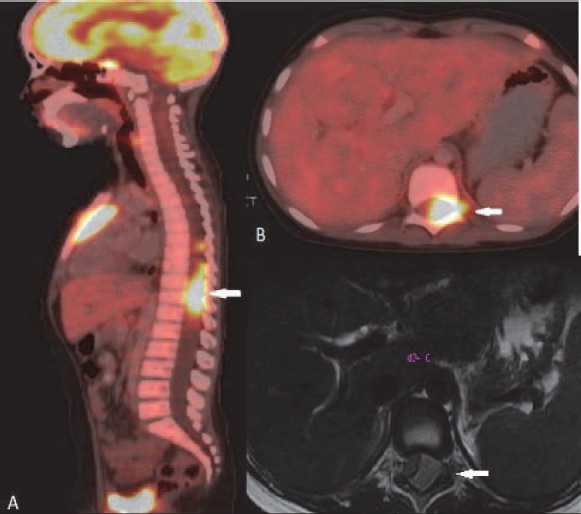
Same patient as in Figure 19. (A) Sagittal fused PET/CT image shows FDG avid intraspinal deposit. Axial fused PET/CT images (B) and axial T2W MRI image (C) confirm the epidural location of the lesion
